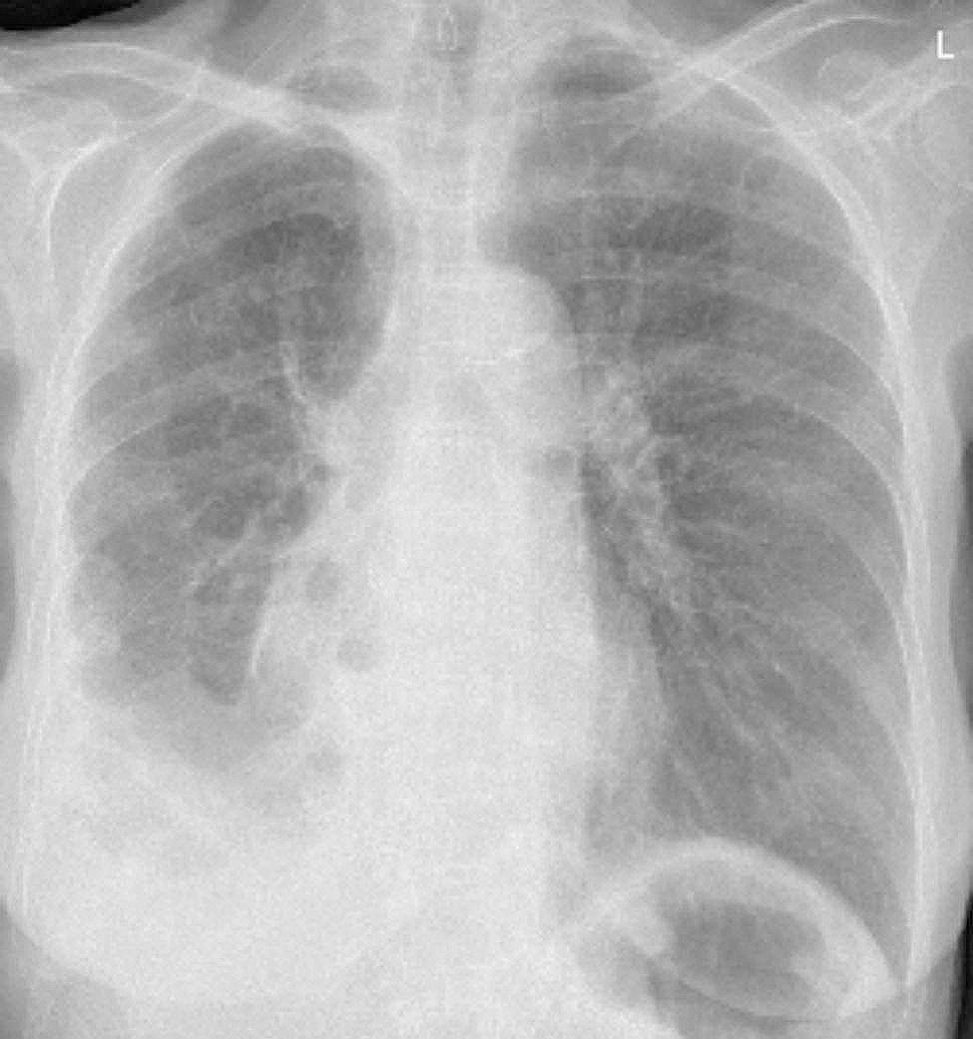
Her chest x-ray after her third operation on postoperative day 1
